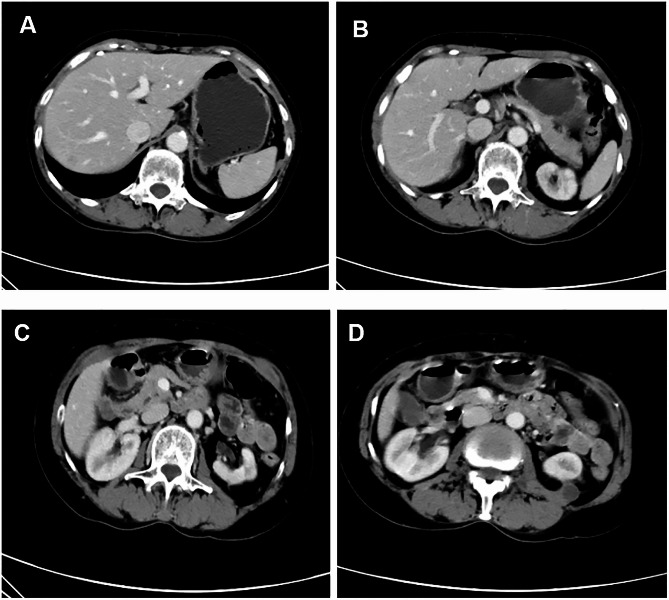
Contrast-enhanced abdominal CT scan after switching to alectinib. Panels A-D: Two months after switching the therapy to alectinib, showing no recurrence or enlargement of cystic lesions in the kidneys and liver
